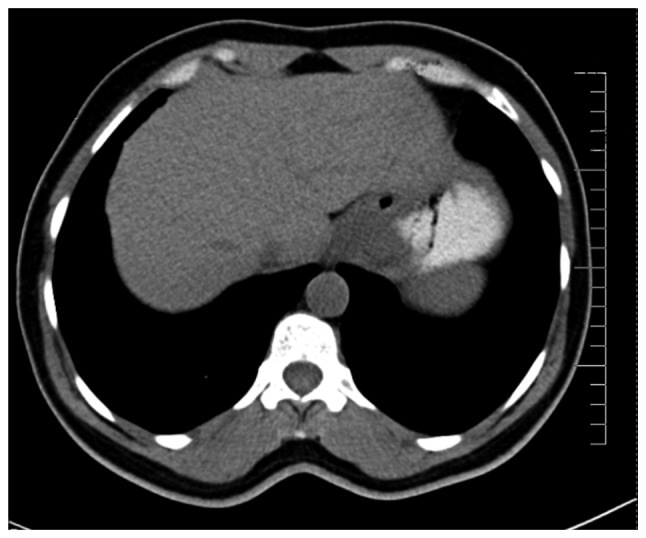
Computed tomography scan revealing an esophageal gastrointestinal stromal tumor measuring 7×5×3 cm.
